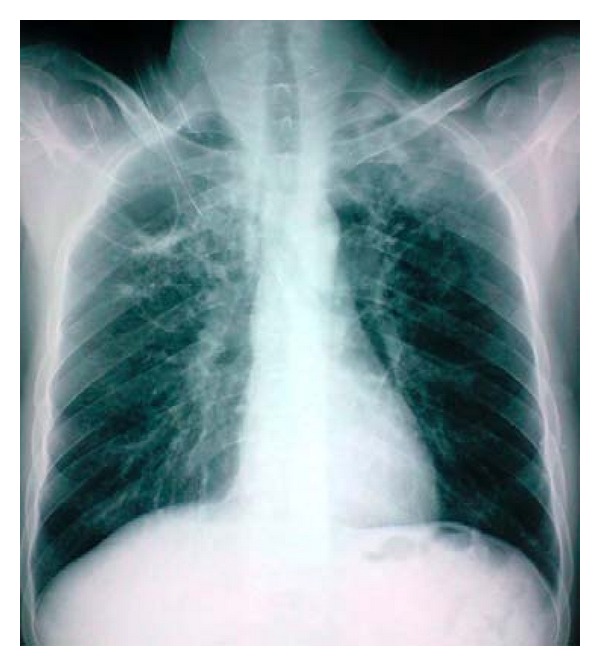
Thoracic X-ray revealed bilateral nonhomogenous infiltrations and cavitary lesions in the right lung.
